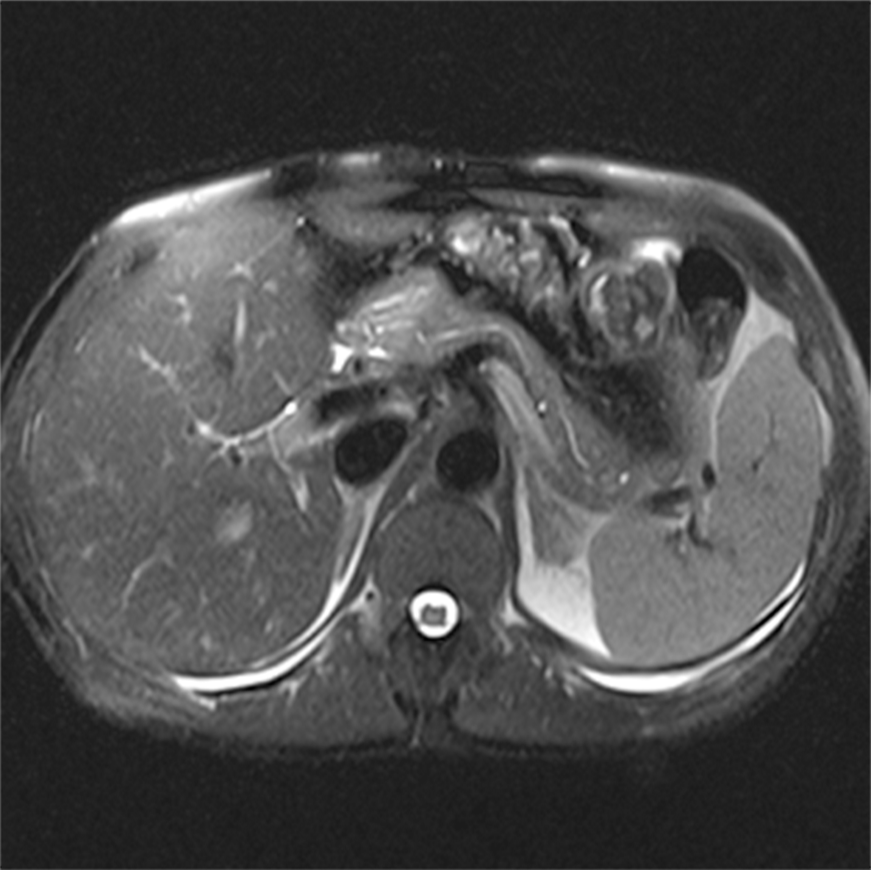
Abdominal MRI (T2-weighted sequence with fat saturation, axial slice), showing two small cysts, one in the tail and one in the transition between body and tail of pancreas.
